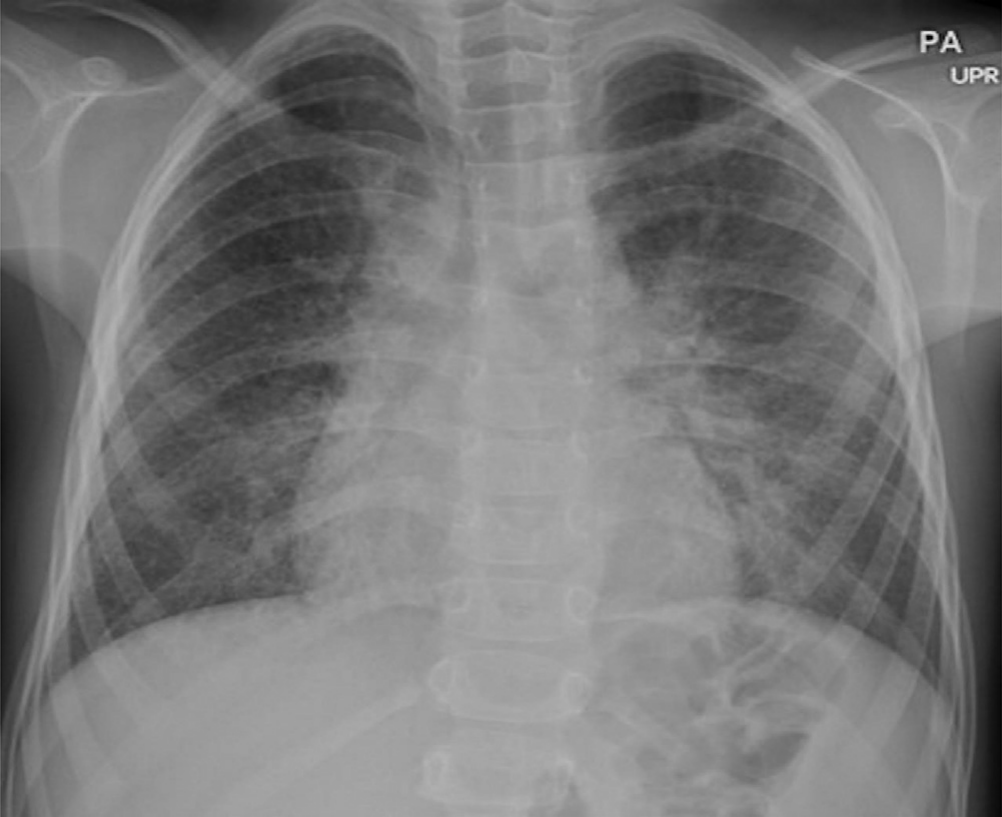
At 5 years of age (case 1), PA chest radiography shows peribronchovascular thickening with bilateral diffuse interstitial thickening.
